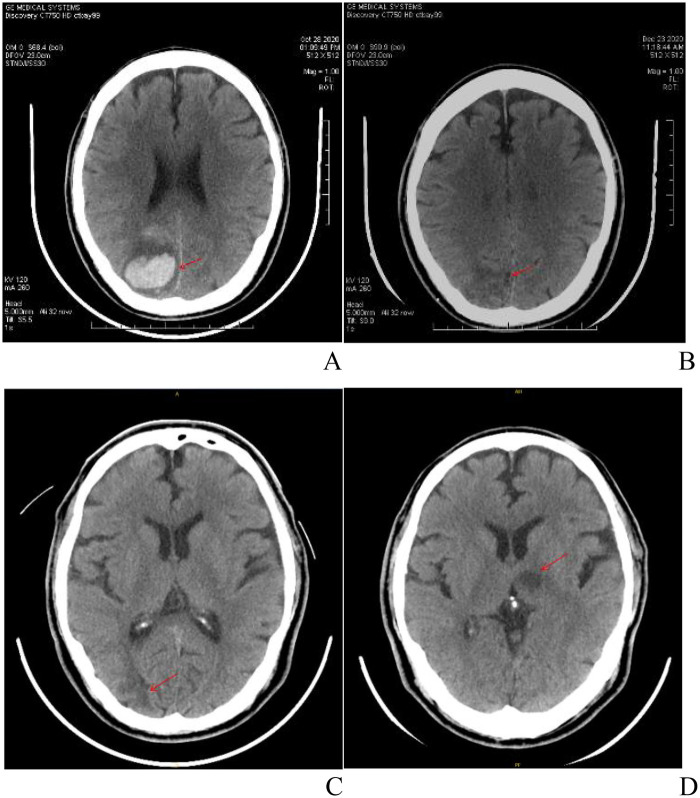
Brain CT of patient with stroke. (A) Right parieto-occipital lobe hemorrhage (volume: approximately 15 mL). (B) Right parieto-occipital lobe hemorrhage was absorbed, and a local soft focus formed. (C) Soft focus in the right parieto-occipital lobe, suspicious low-density shadow in the right semiovoid center. (D) Left thalamo-cerebral peduncle infarction, right parieto-occipital encephalomalacia, and right hemispheric center suspicious hypodensity roughly anterior (arrow).
